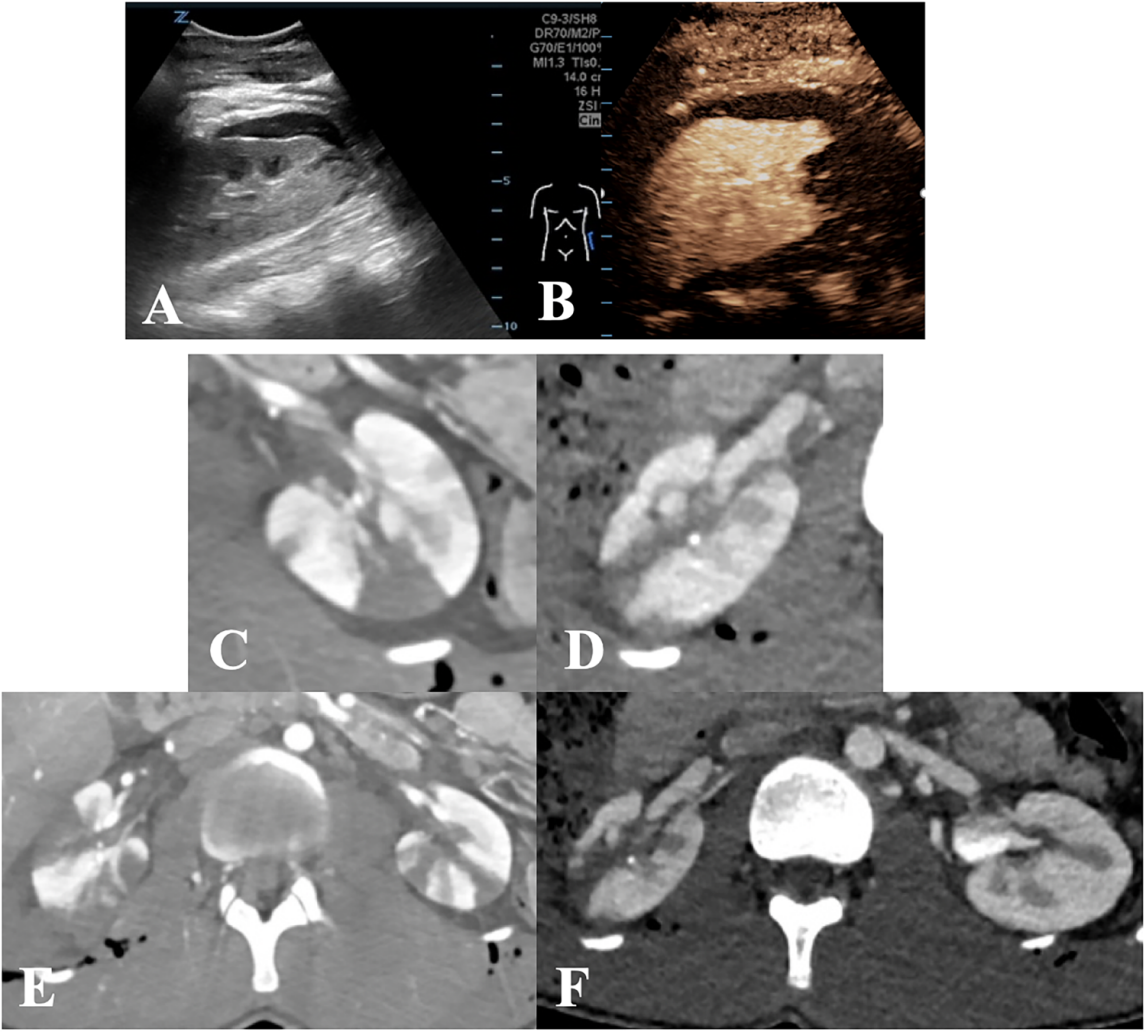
A, B Eight-year-old male, bicycle accident with a sport utility vehicle (SUV). A Laceration and fracture of the kidney (AAST III) and spleen (AAST IV). B Contrast-enhanced ultrasound (CEUS) in the intensive care unit because of instability for transport to the CT unit. 0.8 mL SonoVue applied. Conservative management without complications. C–F Seventeen-year-old male, motorcycle accident. Severe spinal trauma with paraplegia. Temporary vascular segmental lesions of both kidneys, laceration of the right kidney. No urinoma
